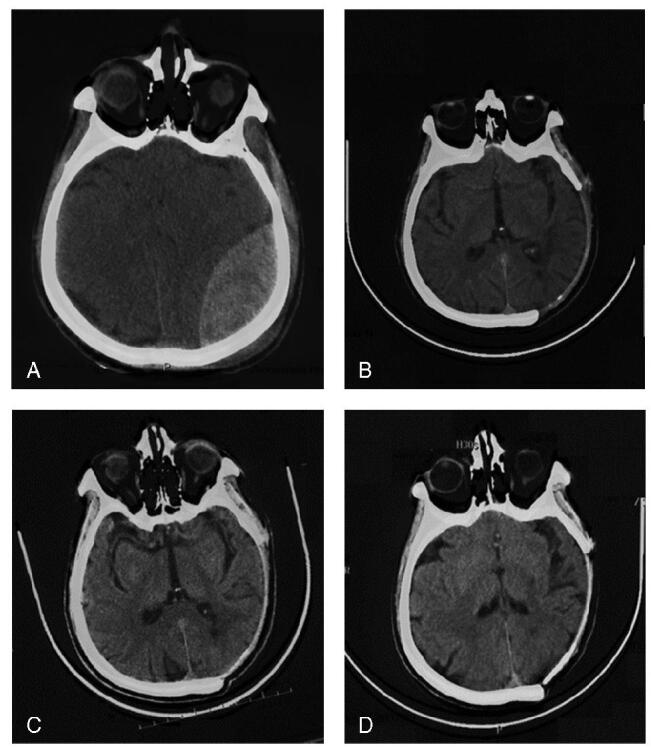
Figure 1. Heterotopic ossification (HO) progression. (A) CT on the day of injury showed a large extradural hematoma in the left tempo-parietal-occipital region; (B) CT at 145 days after DC showed the skull “island” regeneration in the defect area; (C) CT at 171 days after DC showed that the “island” skull regeneration expanded and fused to form a flake regeneration area; (D) CT at 23 months after DC showed the regenerated skull completely covers the defect area. CT = computed tomography, DC = decompressive craniectomy.
